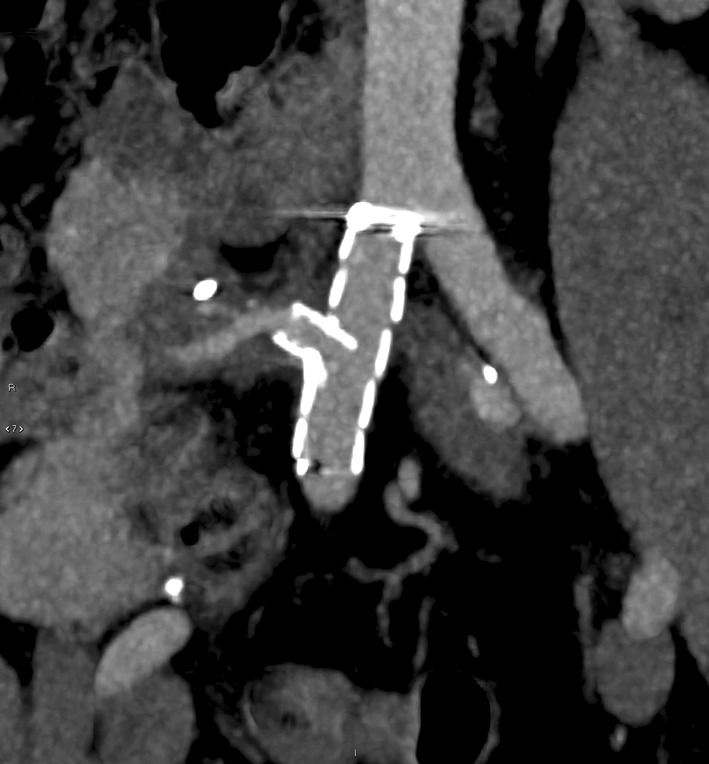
Contrast enhanced CT at 11 months demonstrating a patent graft pancreatic artery and involution of the pseudoaneurysm
